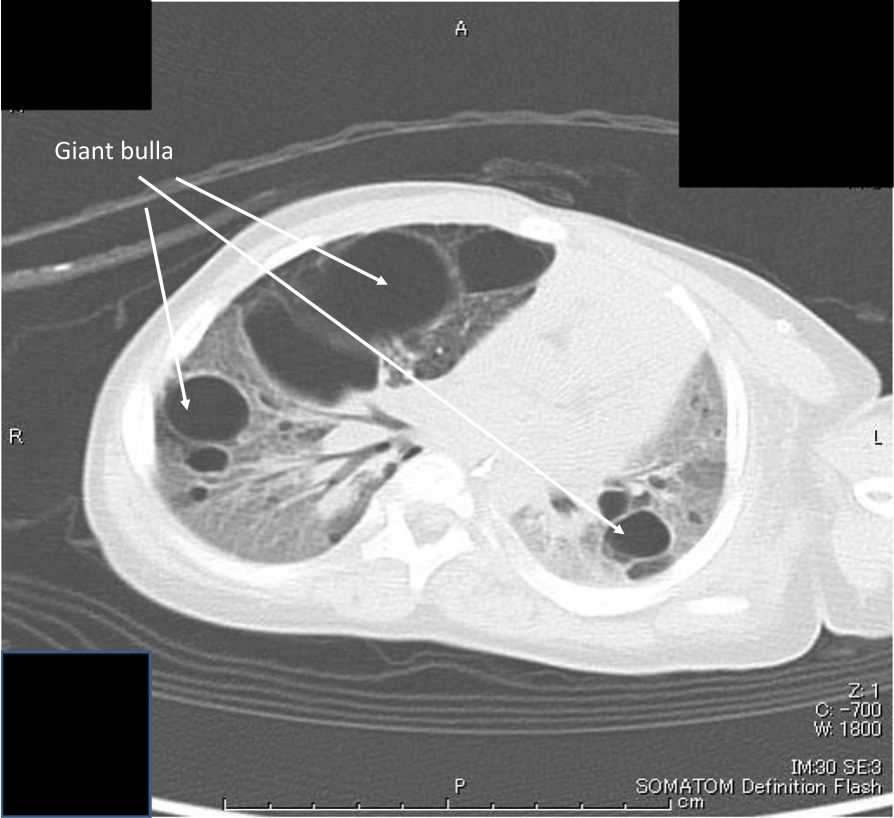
A chest CT at healthy status. Several giant bullae in the bilateral lungs were observed
